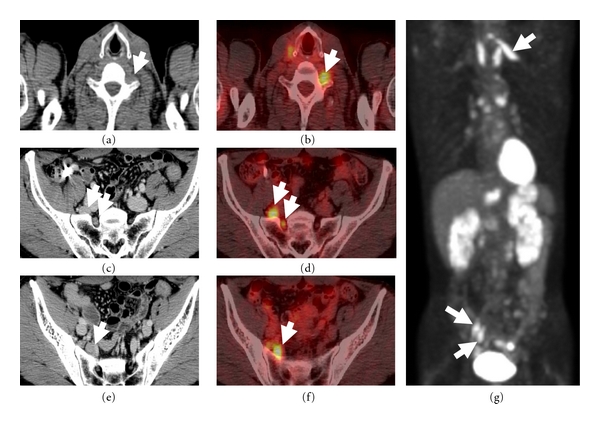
CT (a, c, and e) and FDG-PET (b, d, and f: fusion images; g: coronal MIP image) of whole body in 58-year-old man (case 5). CT shows soft tissue density around the left C6 (a; arrow), right L5 (c; arrows), and S1 nerves (e; arrow). High FDG uptakes are identified as perineural masses (b, d, f, and g; arrows).
